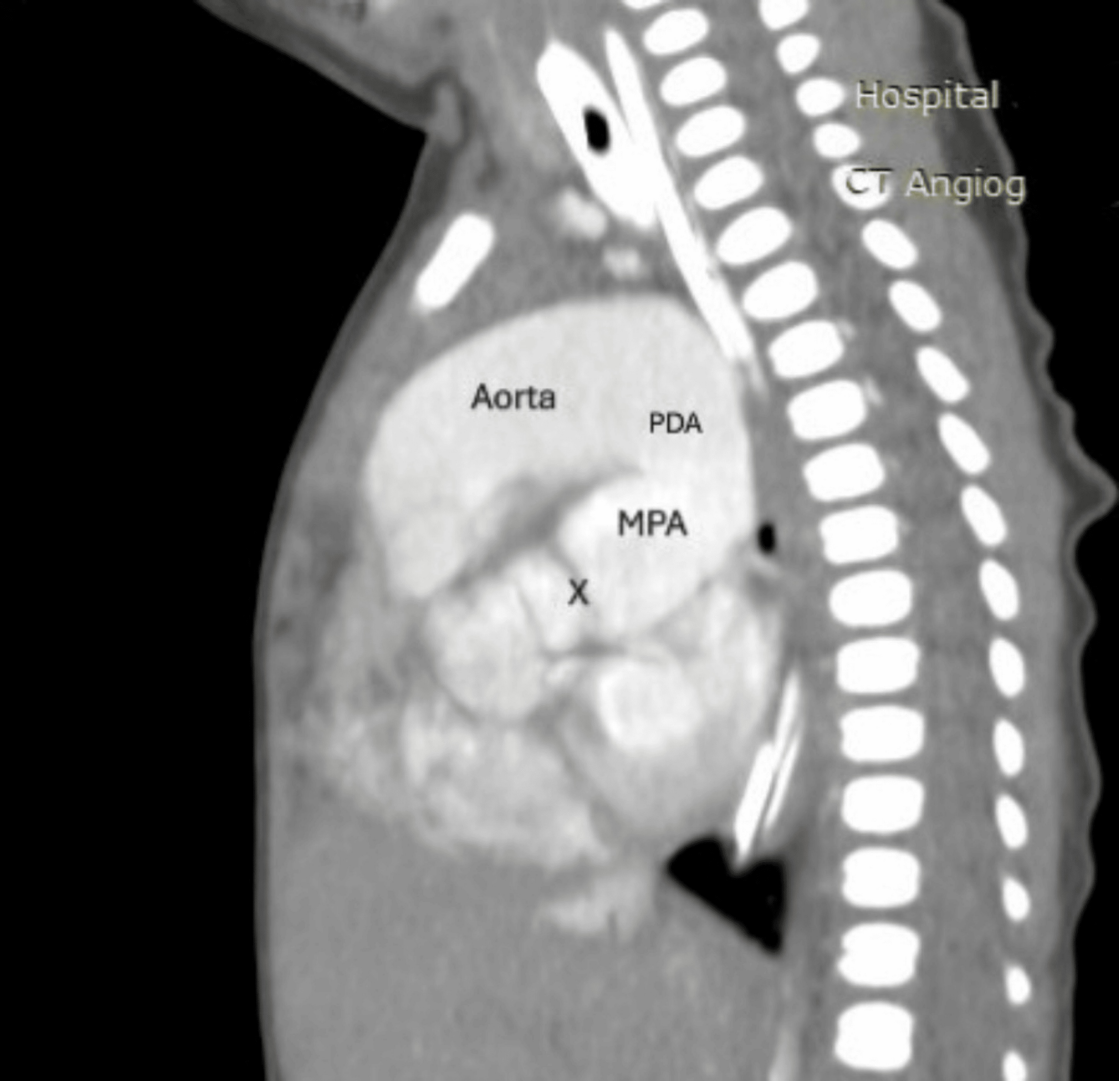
Sagittal contrast-enhanced CT angiography shows the dilated main pulmonary artery (MPA) positioned posterior to the transposed aorta, with a prominent patent ductus arteriosus (PDA) connecting to the descending aorta. The pulmonary valve is labeled as X.
